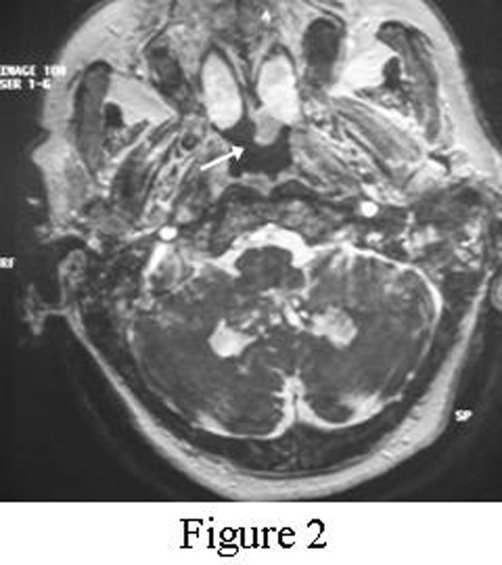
Axial MRI T2 weighted sequence in which a hypodense lesion is seen on the posterior end of the left inferior turbinate (white arrow).
